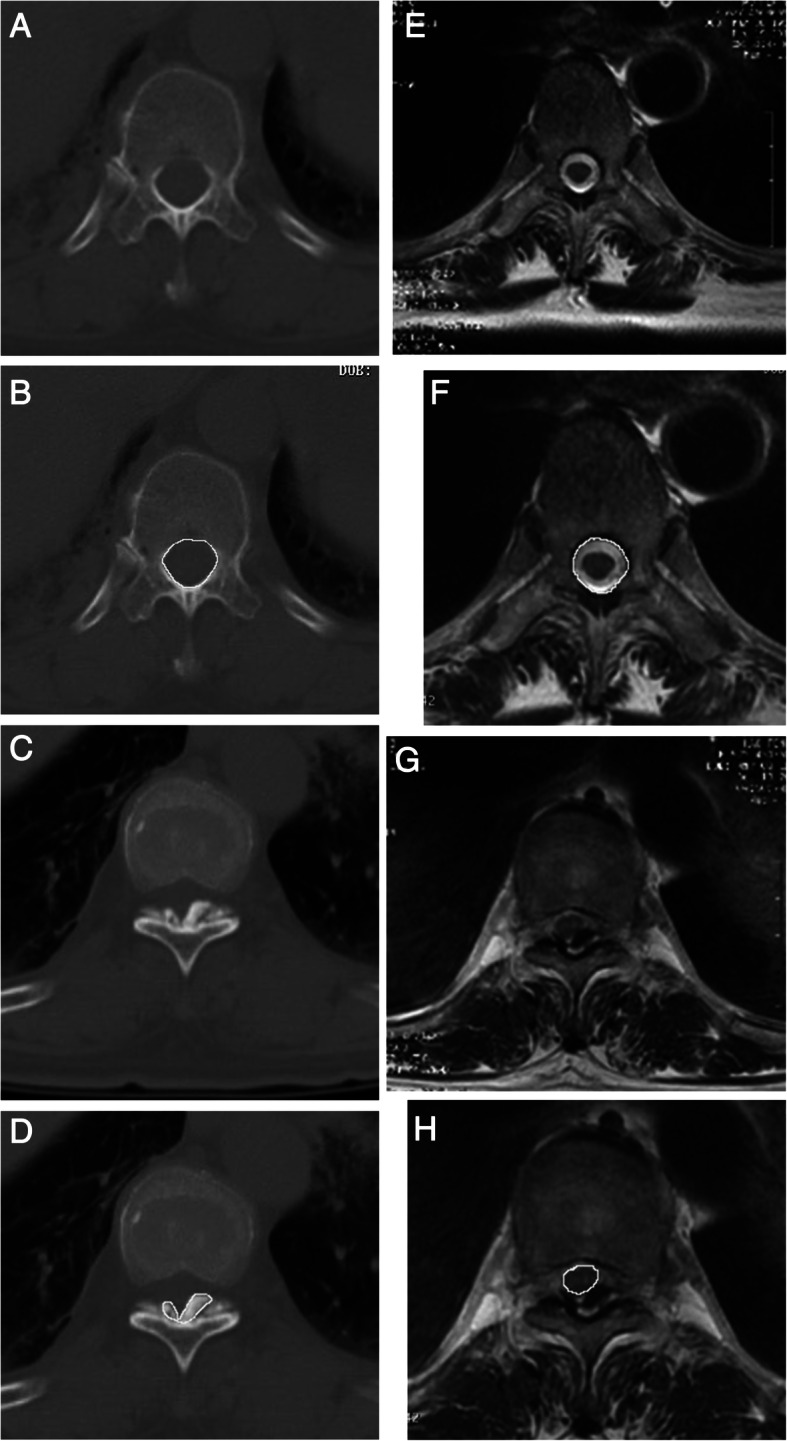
Radiographic measurement. (A) Normal spinal canal at the pedicle level of the maximum compression segment. (B) Cross-section area (CSA) of the normal spinal canal. (C) Axial view of computed tomography scan at the segment of maximum compression. (D) CSA of the ossified mass. (E) Magnetic resonance imaging (MRI) at the pedicle level. (F) Measurement of CSA of the dural sac at the pedicle level. (G) MRI at the segment of maximum compression. (H) CSA of the spinal cord
